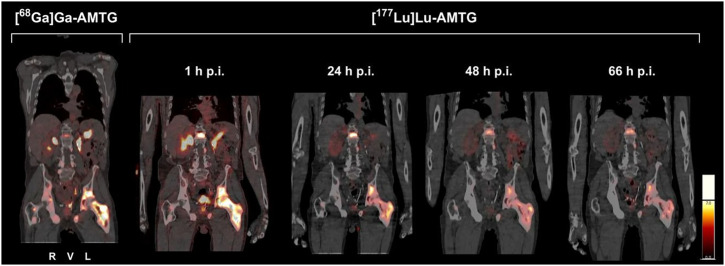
Uptake of [^68^Ga]Ga-AMTG and [^177^Lu]Lu-AMTG in 70-y-old patient with mCRPC with insufficient PSMA uptake and exhausted treatment options. Coronal section of pretherapeutic [^68^Ga]Ga-AMTG PET/CT image (left) displays pronounced uptake in osseous metastases, including in ilium and femoral neck and shaft. Intratherapeutic [^177^Lu]Lu-AMTG SPECT/CT image (right) at 1, 24, 48, and 66 h after injection shows intense and persistent activity retention in these lesions. All images were identically scaled to SUVs. p.i. = postinjection.
